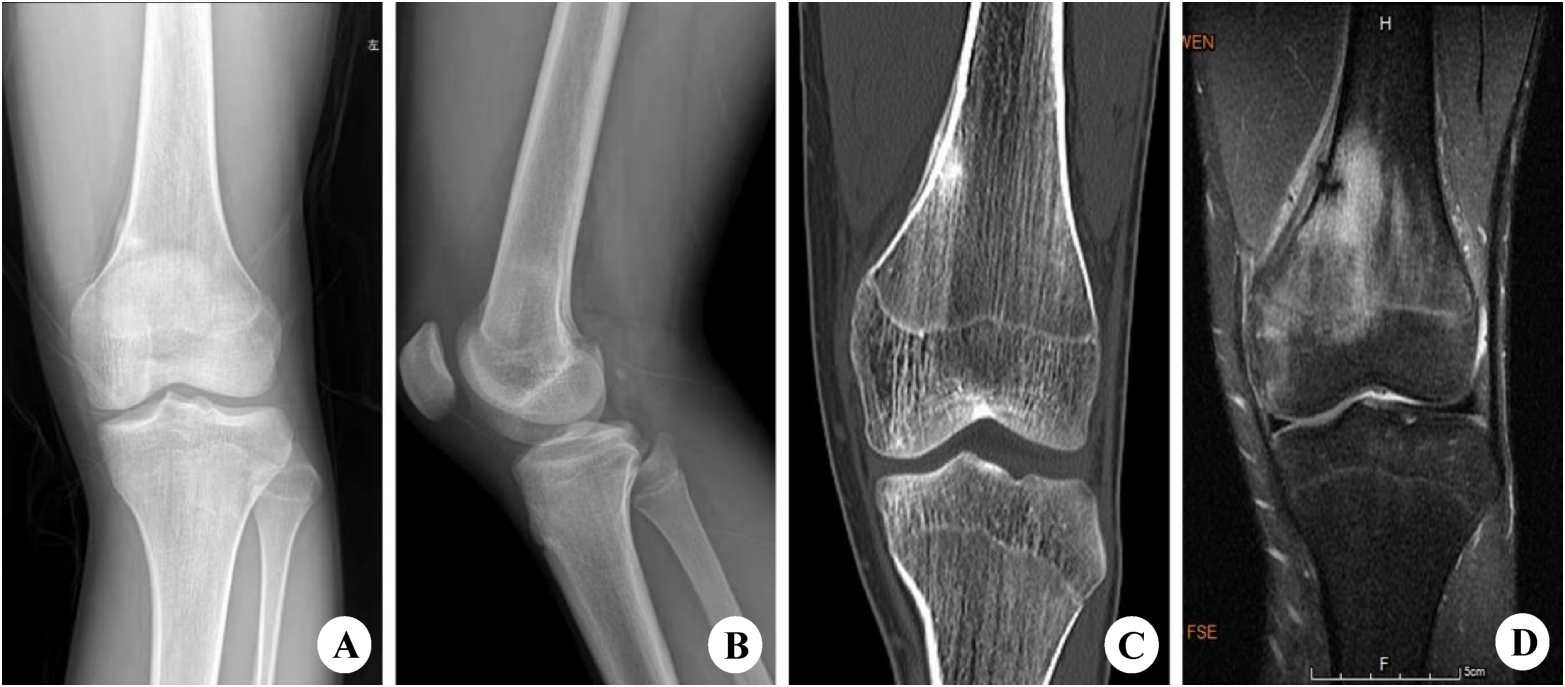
A 20-year-old male with a left femoral Type I stress fracture. (A,B) On the anteroposterior and lateral x-ray views, increased cortical density is observed in the distal region of the left femur. (C) CT scan reveals discontinuity of the cortical bone with strip-like high-density shadows along the edges in the distal region of the left femur. (D) MRI shows signs of fracture line at the distal end of the left femur, accompanied by large areas of long T1 and long T2 signal intensity in the bone marrow cavity.
